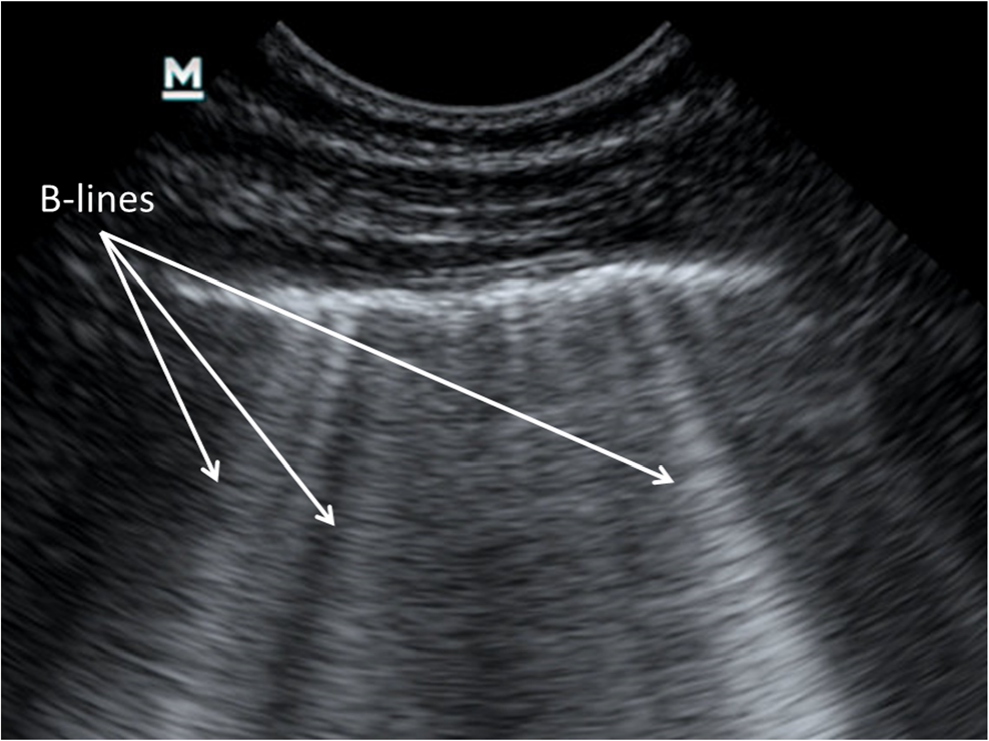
An ultrasound image from the left anterior lower lung zone in a 2-year-old boy who presented with symptoms of pneumonia shows multiple and confluent B-lines in a single view representing an interstitial disease pattern
